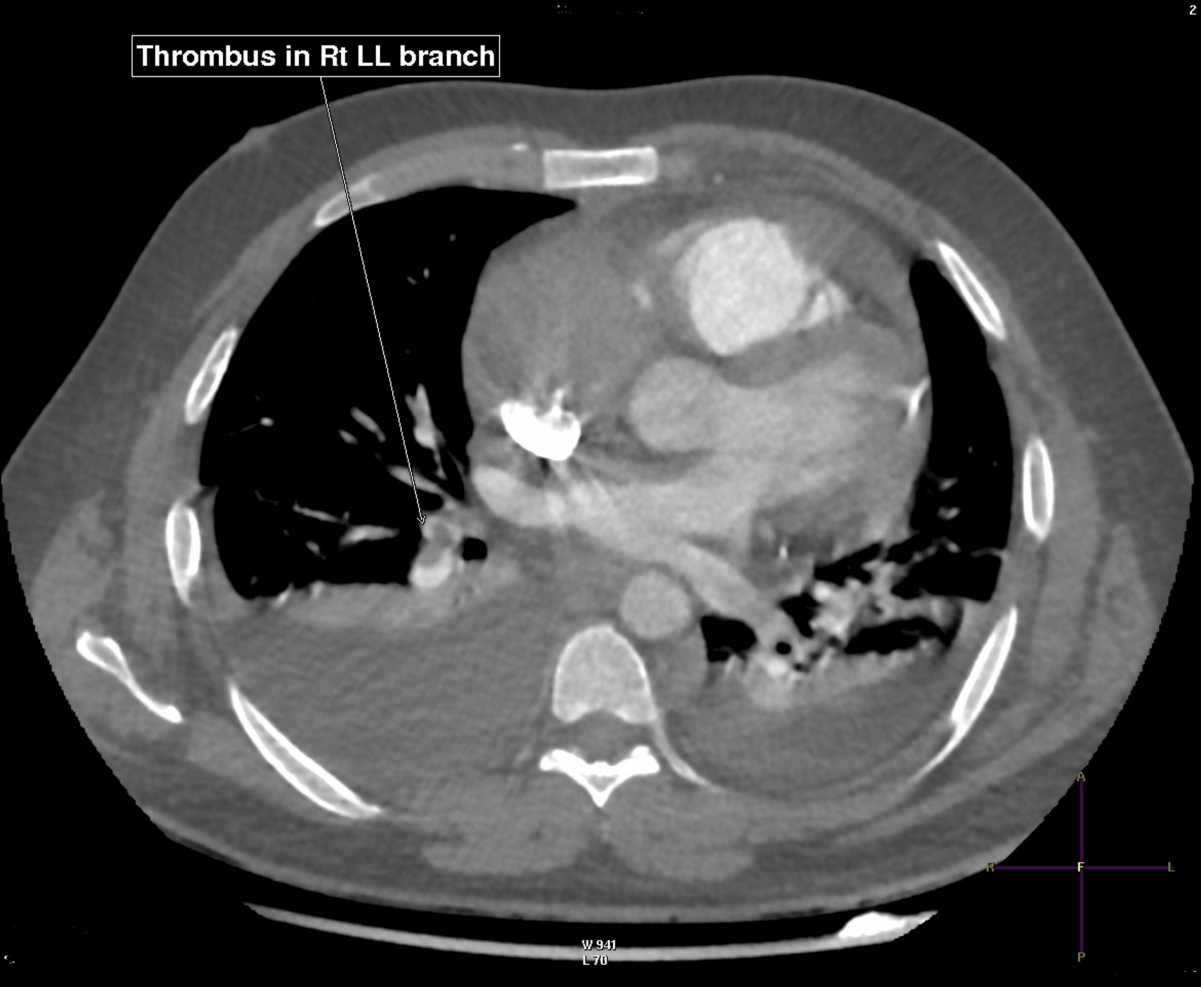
Computed tomography (CT) angiography showing thrombus in the right lower lobe (Rt LL) branch
